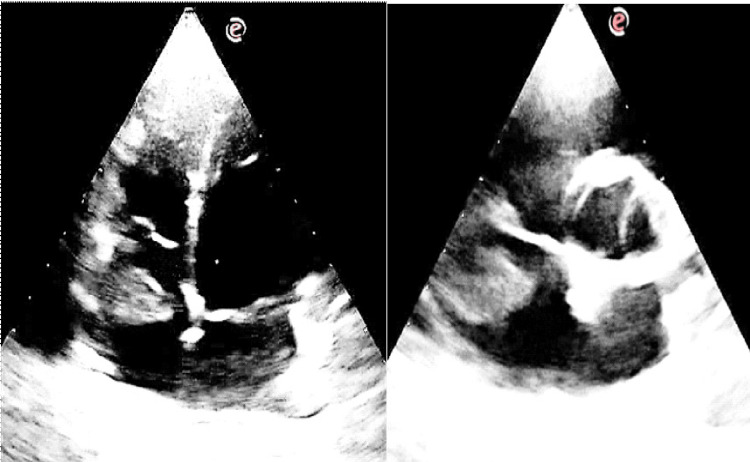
Two-dimensional echocardiography apical four-chamber view showing echogenic mass in the right atrial (RA) attached to the free wall (left) and modified parasternal short axis view showing mass in the RA (right).
